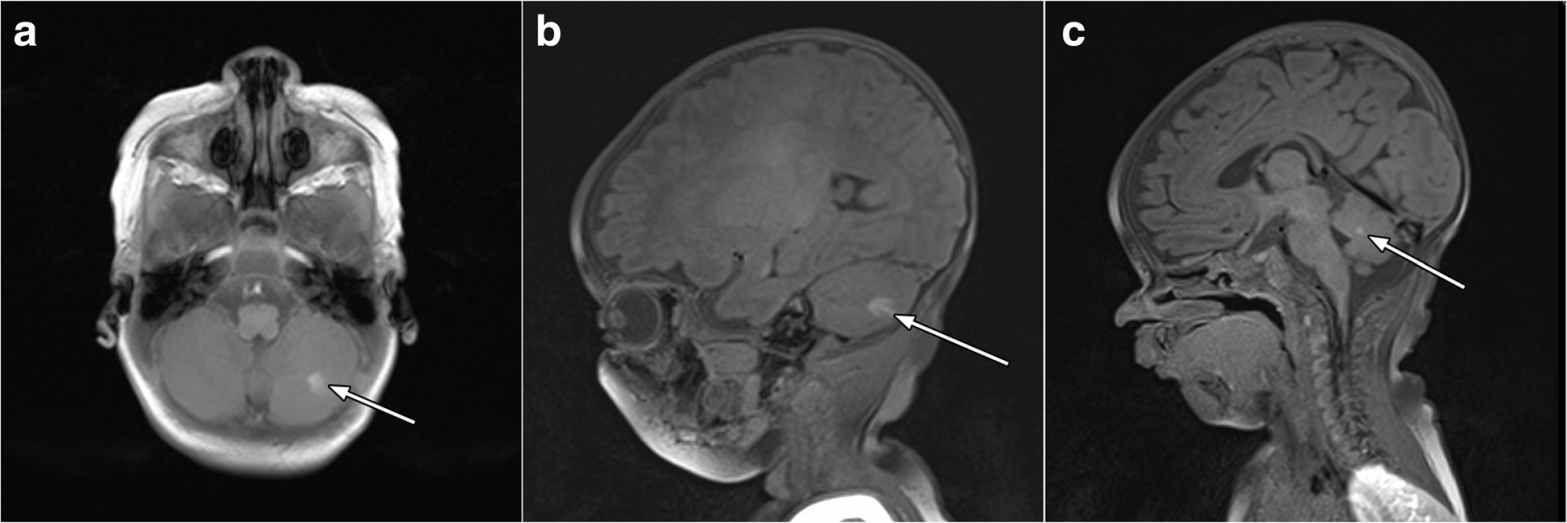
Magnetic resonance imaging shows the presence of melanin in the structure of the brain. a Postcontrast axial T1-weighted (W) MRI and (b) postcontrast sagittal T1W image with focus on the altered MR signal in the left hemisphere of the cerebellum is determined. c Sagittal T1W image with focus on the abnormal MR signal on the cerebral pia mater of the cerebellum. The imaging findings described were diagnostic for neurocutaneous melanosis
